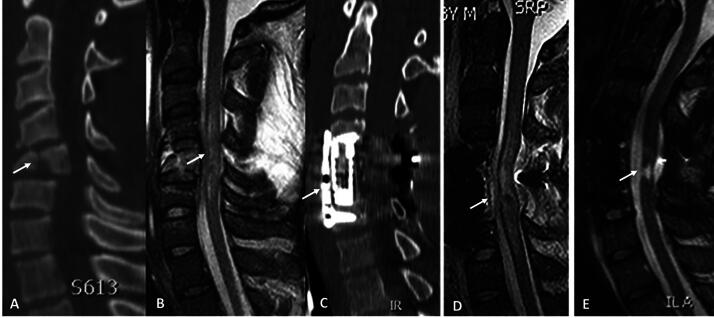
Index case. Midsagittal CT cuts indicating C5 tear-drop fracture (arrow) with translation of C5 body into spinal canal (A). Midsagittal T2-weighted MRI indicating reduction with hematomyelia and spinal cord swelling (arrow). Intramedullary lesion length (IMLL) was 49.7 mm (B). C5 corpectomy and circumferential C4-C6 fusion was conducted (arrow) (C). Immediate post-operative midsagittal T2-weighted MRI indicating spinal cord decompression (arrow) and IMLL of 62.7 mm (D). Midsagittal T2-weighted MRI 6 months following trauma indicating a small syrinx (arrow) at C5 level (E).
